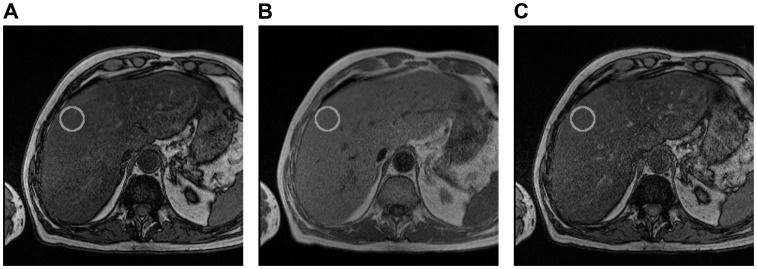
Axial magnetic resonance images of a patient with a typical ROI (solid grey circle) used for fat analysis. A) 2.38 ms (opposed phase), B) 4.76 ms (in phase), C) 7.14 ms (opposed phase).
